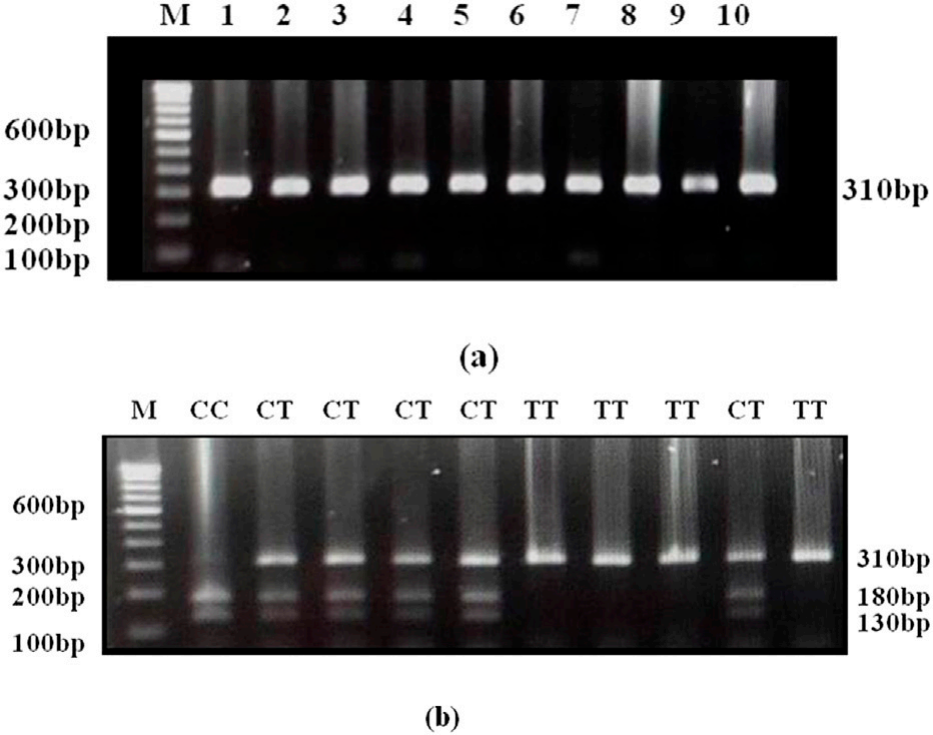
(a) Gel picture showing amplicon of 310bp of DRD2 Taql. (b) Gel picture of Taql digested DRD2 PCR products. Homozygous wild (CC): 180 and 130bp; Heterozygous (CT): 310, 180 and 130bp; Homozygous mutant (TT): 310bp (remains intact).
